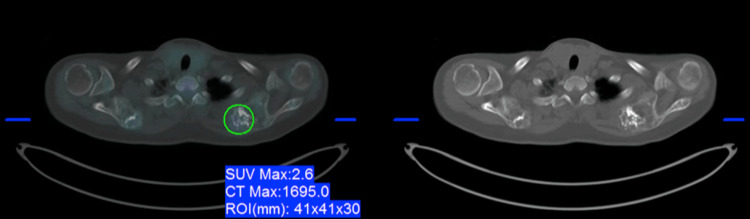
CT and fused PET/CT axial images of the superior thoracic region showing an expansile cartilaginous lesion in the left scapula demonstrating low-grade uptake (SUV: 2.6) CT: computed tomography, PET: positron emission tomography, SUV: standardized uptake value
